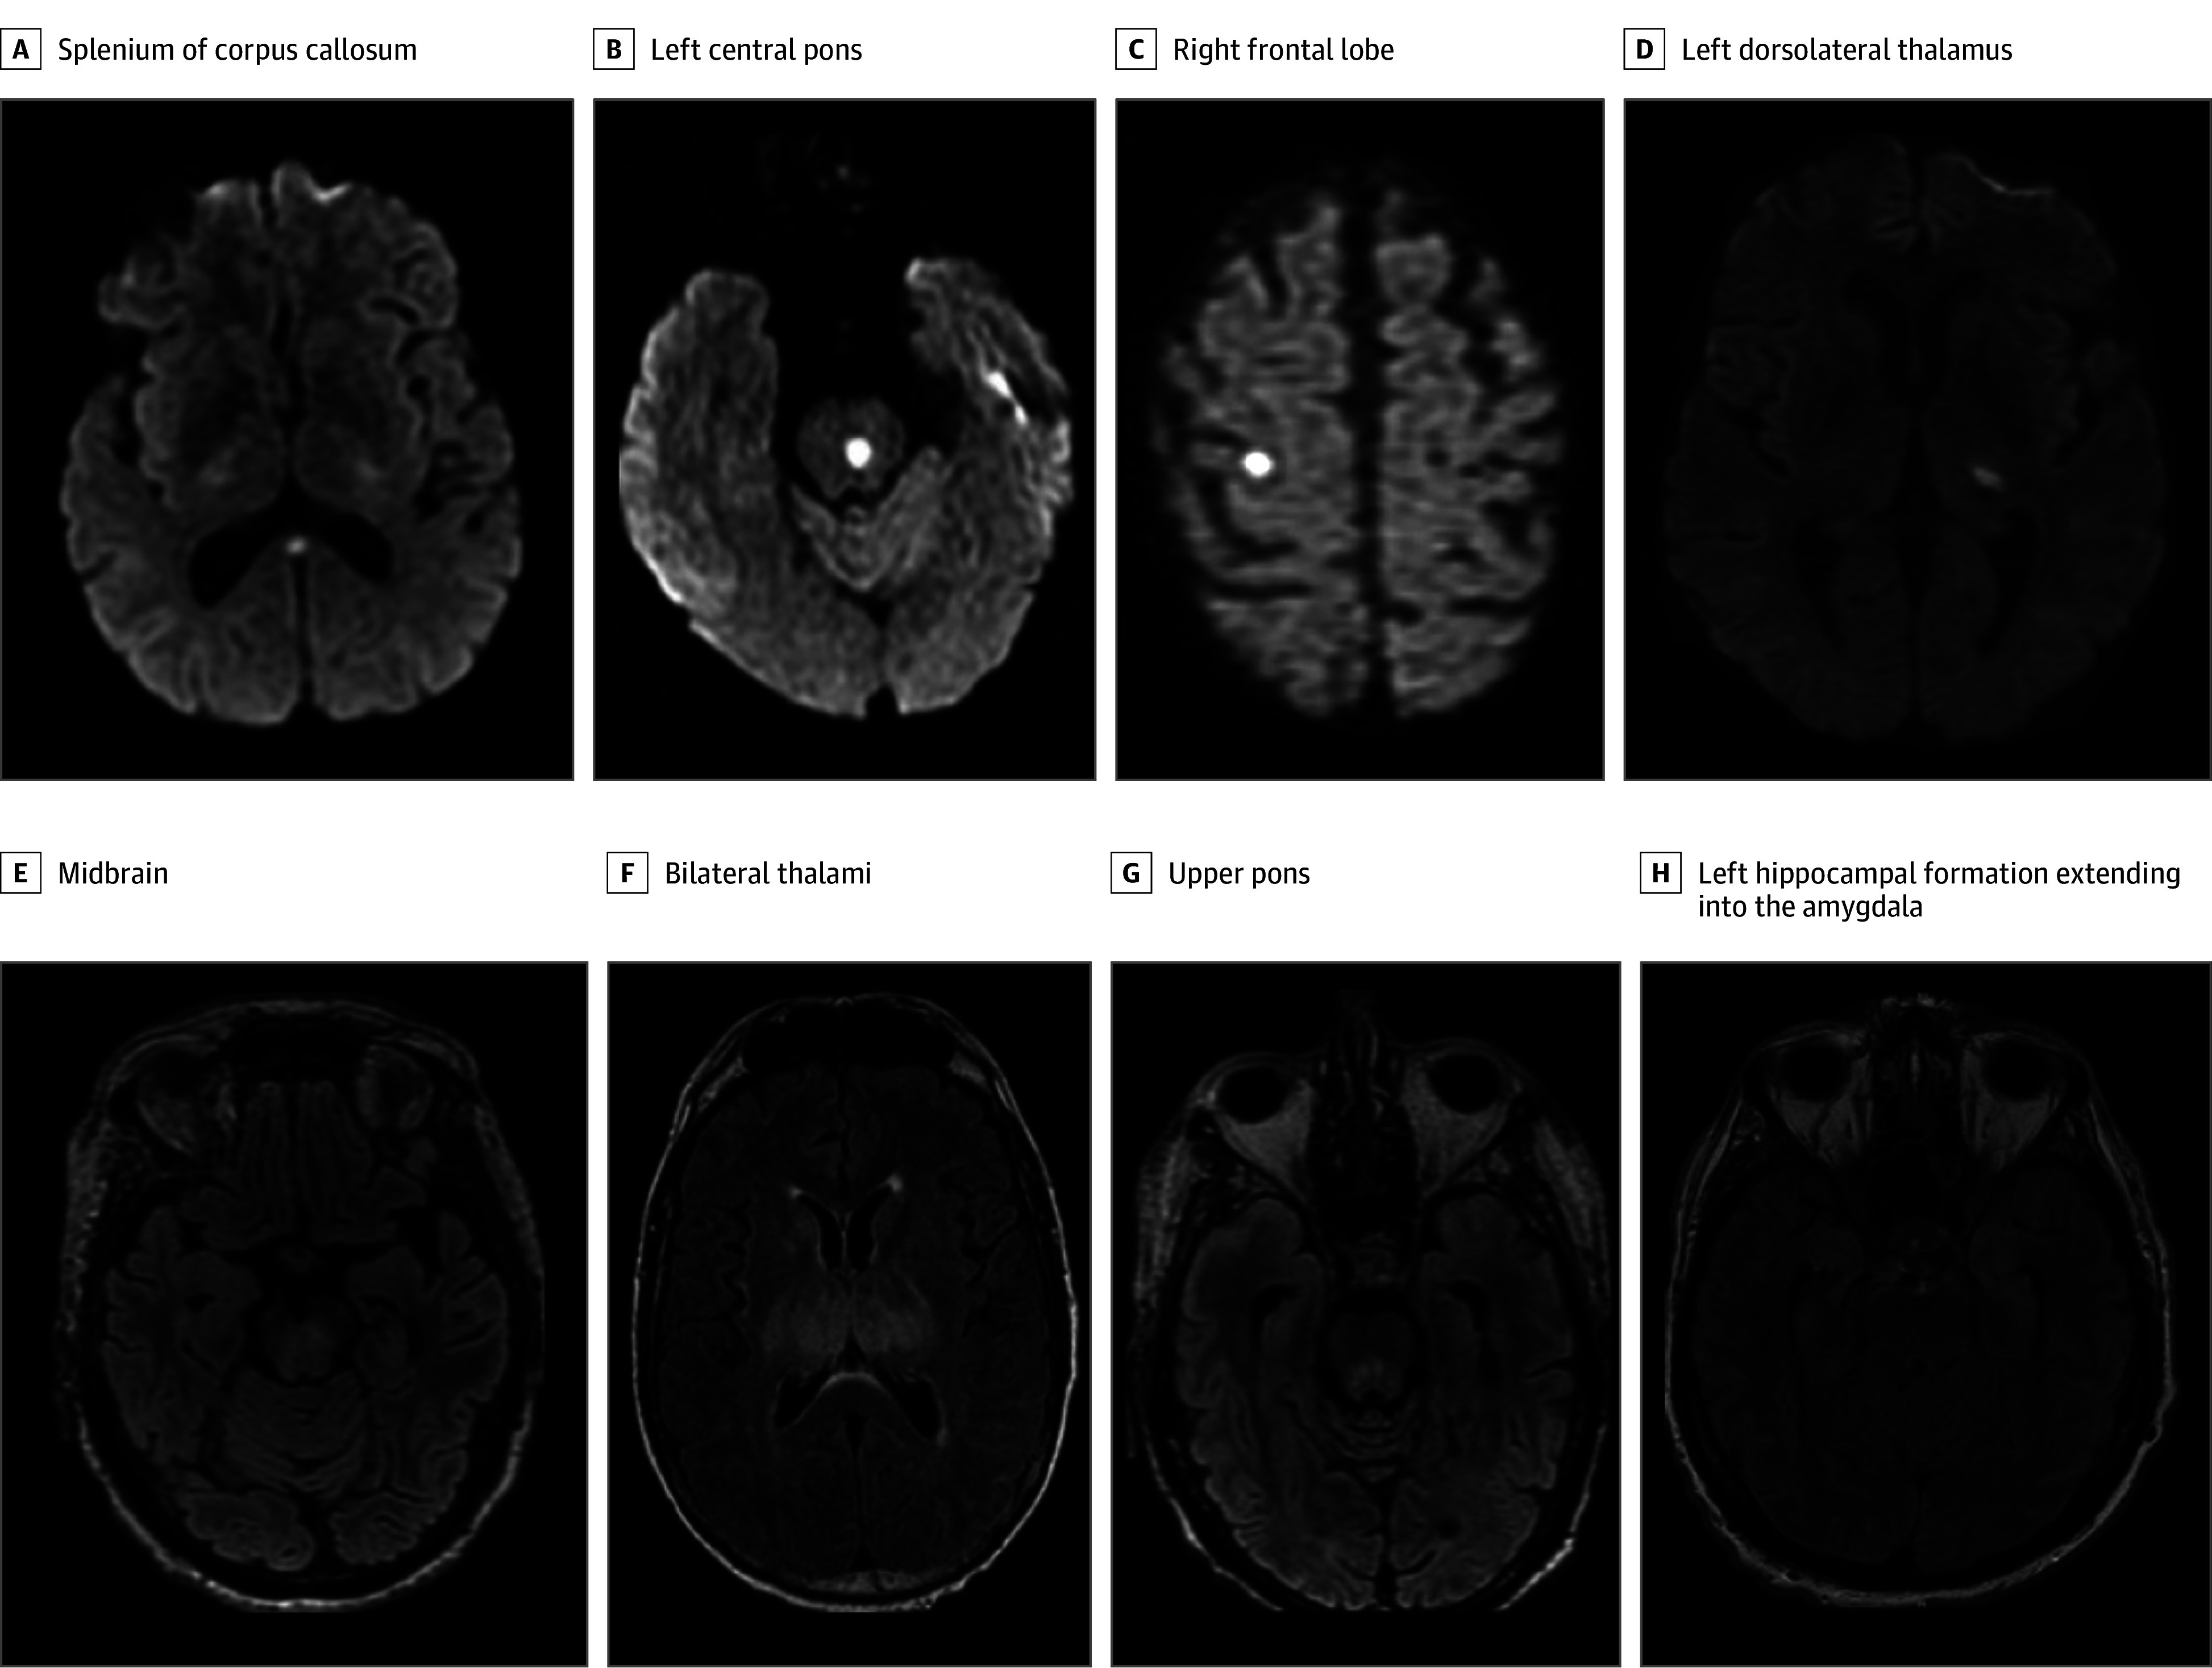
Diffusion-Weighted Imaging Brain Magnetic Resonance Imaging Sequences From Different Patients Showing Focal Areas of Diffusion Restriction A-D, Patients had an apparent diffusion coefficient correlate with decreased signal suggesting acute ischemia. E-G, Fluid-attenuated inversion recovery images of various patients showing areas of subtle increased fluid attenuated inversion recovery signal.
